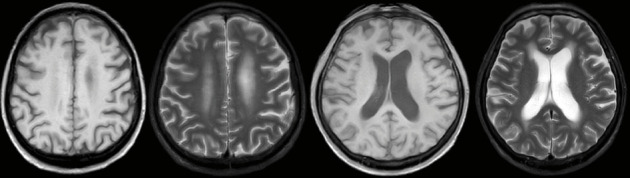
Brain magnetic resonance imaging (MRI) scan upon admission.
